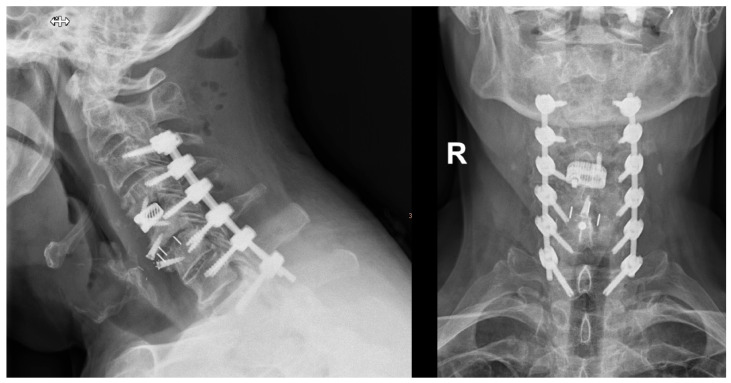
Postoperative X-ray after implantation of pedicle screws from C3-T1. In this specific case, spondylitis several years after anterior cervical discectomy and fusion with implant loosening of the upper cage at C4/5 made revision surgery necessary with implantation of a new cage at C4/5, and subsequent posterior instrumentation. R = right.
